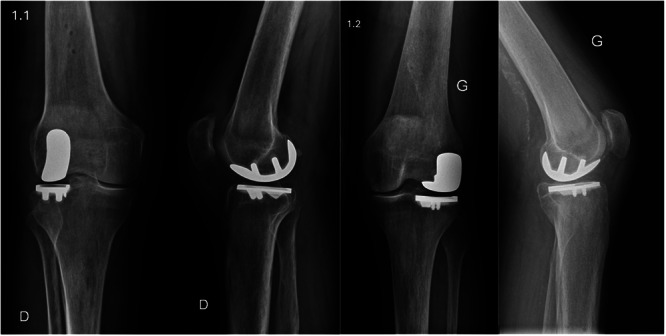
Implant designs: (1.1) Post‐operative anteroposterior and lateral radiographs of a right knee following IBRA‐assisted UKA with the MAKO system. (1.2) Post‐operative anteroposterior and lateral radiographs of a left knee following ILRA‐assisted UKA with the NAVIO system. IBRA, image‐based robotic‐assisted; UKA, unicompartmental knee arthroplasty.
